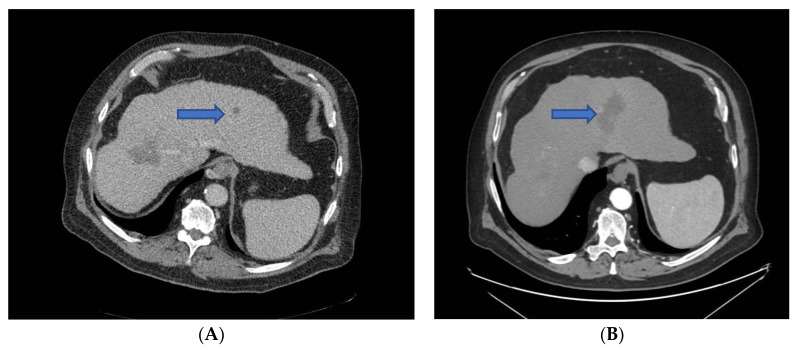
(A,B) show an example of a CT scan in a patient with a left lobar HCC nodule prior to (A) and at 1 month after (B) RFA (blue arrow indicating the HCC nodule).
